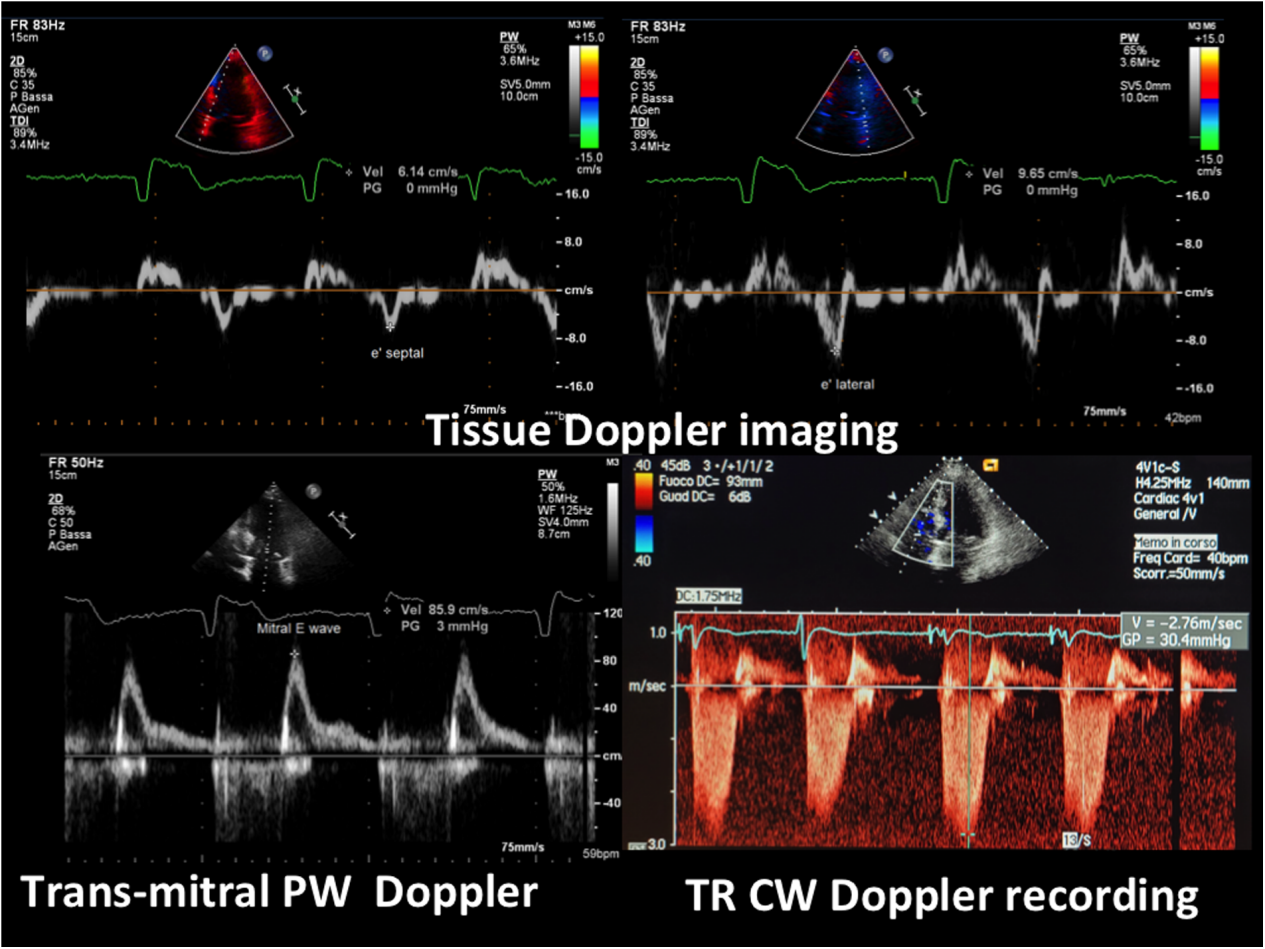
E/e’ and pulmonary pressure evaluation by transthoracic Doppler. At the top, tissue Doppler imaging of the movement of the septal (on the left, e’ septal wave) and of the lateral mitral annulus (on the right, e’ lateral wave); at the bottom (on the left) transmitral blood flow Doppler recording (mitral E wave) (the A wave is lacking since the rhythm is atrial fibrillation). The E/e’ ratio is elevated, indicating a high mean left atrial pressure confirmed by the elevated pulmonary pressure, as assessed by the maximal velocity of the TR jet (>2.7 cm/s) (bottom right). PW = pulsed Doppler; CW = continuous Doppler; TR = tricuspid regurgitation.
